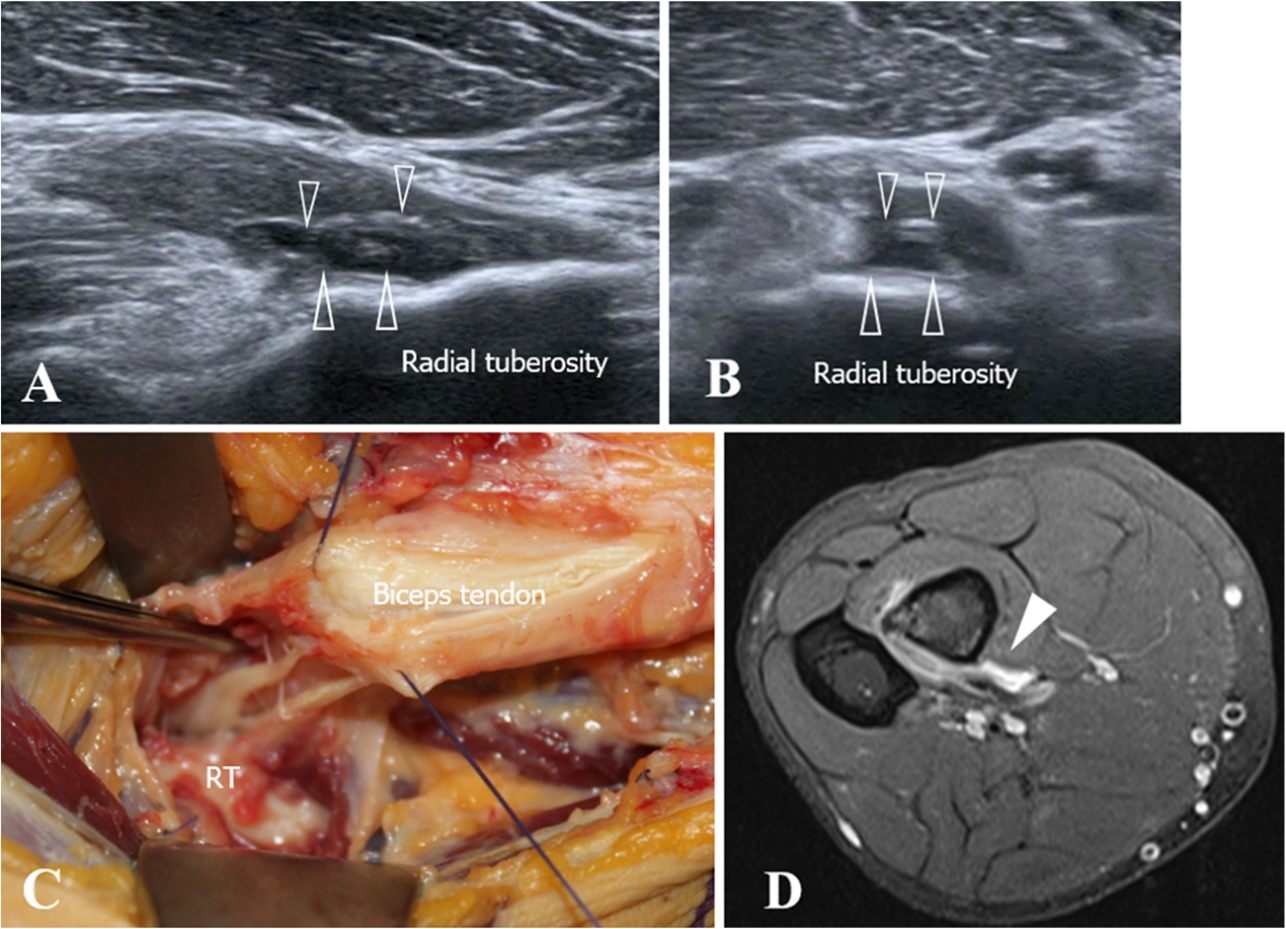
Ultrasound and MRI views of a type 2B injury (high-grade partial tear at the attachment) and image of surgical confirmation. a In the long-axis view, a high-grade partial detachment (*arrowheads*) affecting more than 50% of the DBBT (biceps tendon) is observed adjacent to the radial tuberosity. The most superficial *arrowheads* show a hyperechogenic line that corresponds to the torn tendon interface. The defect percentage is calculated by considering tendon thinning, not by considering the amount of anechoic fluid, as its image can be easily altered owing to transducer pressure over the examination region. b Short-axis view of a. The important anechoic defect demonstrates that most tendon fibres are torn (*arrowheads*). c Surgical image correlating with images a and b. The forceps point to the attachment of some remaining DBBT (biceps tendon) fibres to the radial tuberosity (*RT*). d Elbow MRI, axial PD with FS-weighted sequence. An intratendinous hyperintense signal (*arrow*), similar to that of fluid, shows a tendon defect affecting more than 50% of the DBBT and therefore compatible with a high-grade partial detachment type 2B
